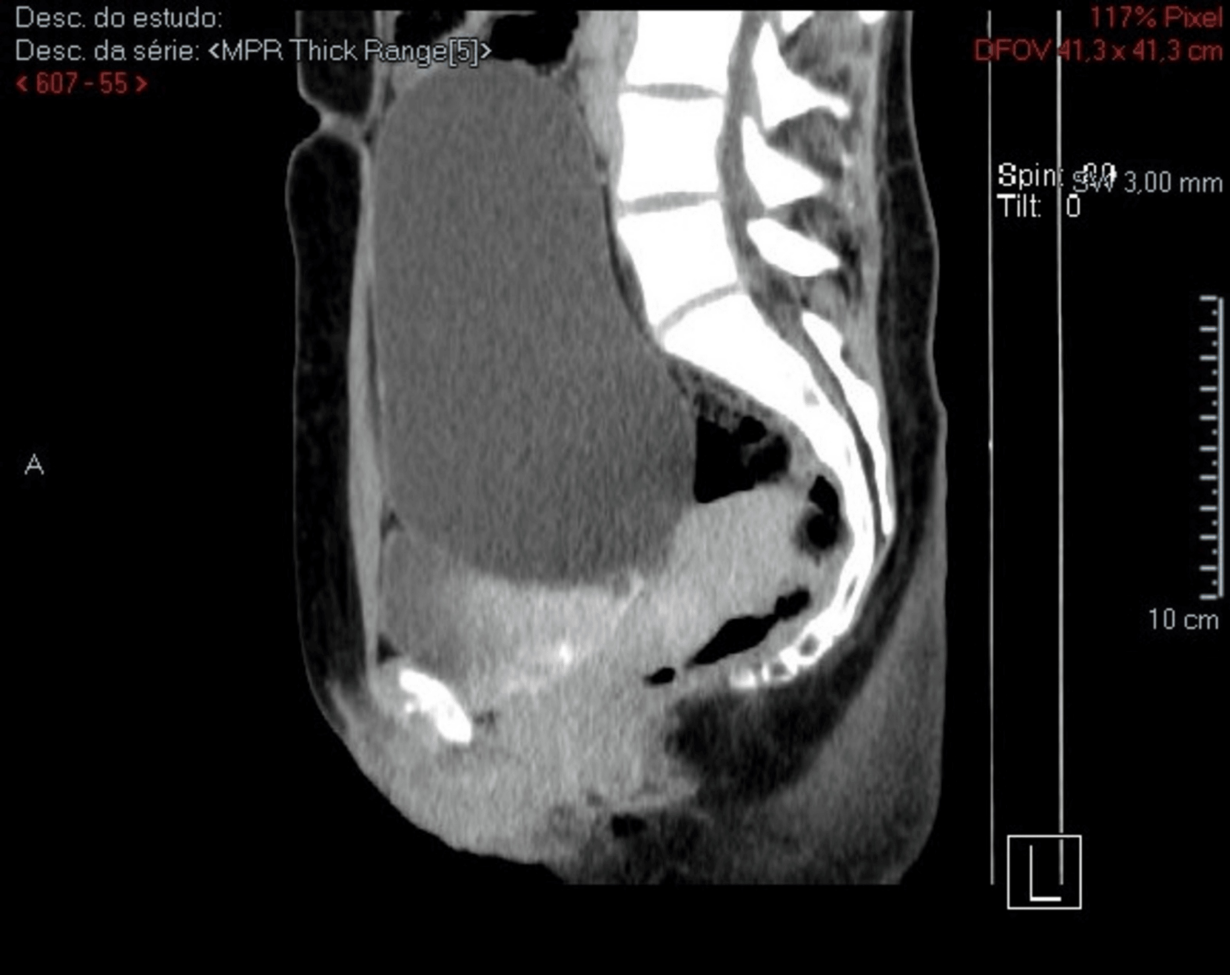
Abdominopelvic CT scan (sagittal cut) This image demonstrates a clear separation between the bladder and the cyst, especially with contrast. CT: computed tomography
